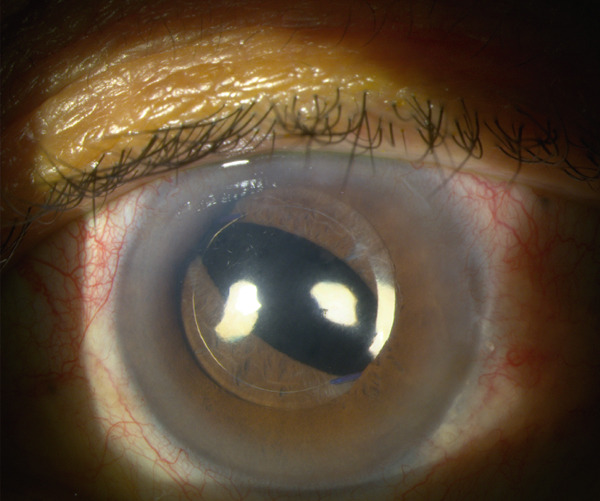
Primary IOL subluxation prior to vitrectomy. CT Lucia 602 hydrophobic acrylic lens with polyvinylidene fluoride (PVDF) monofilament haptics, externalized at 2 o′clock and 8 o′clock positions.
